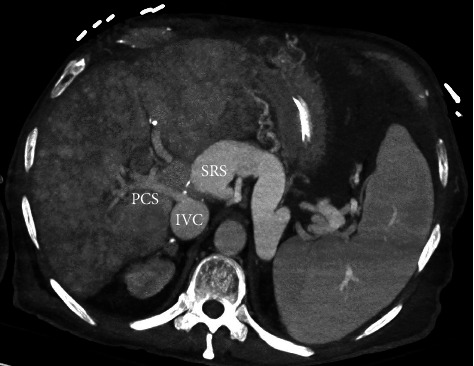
Maximum intensity projection contrast enhanced computed tomography showing the anatomical relationship between the transplant portocaval shunt (PCS), inferior vena cava (IVC), and the massive splenorenal shunt (SRS).
